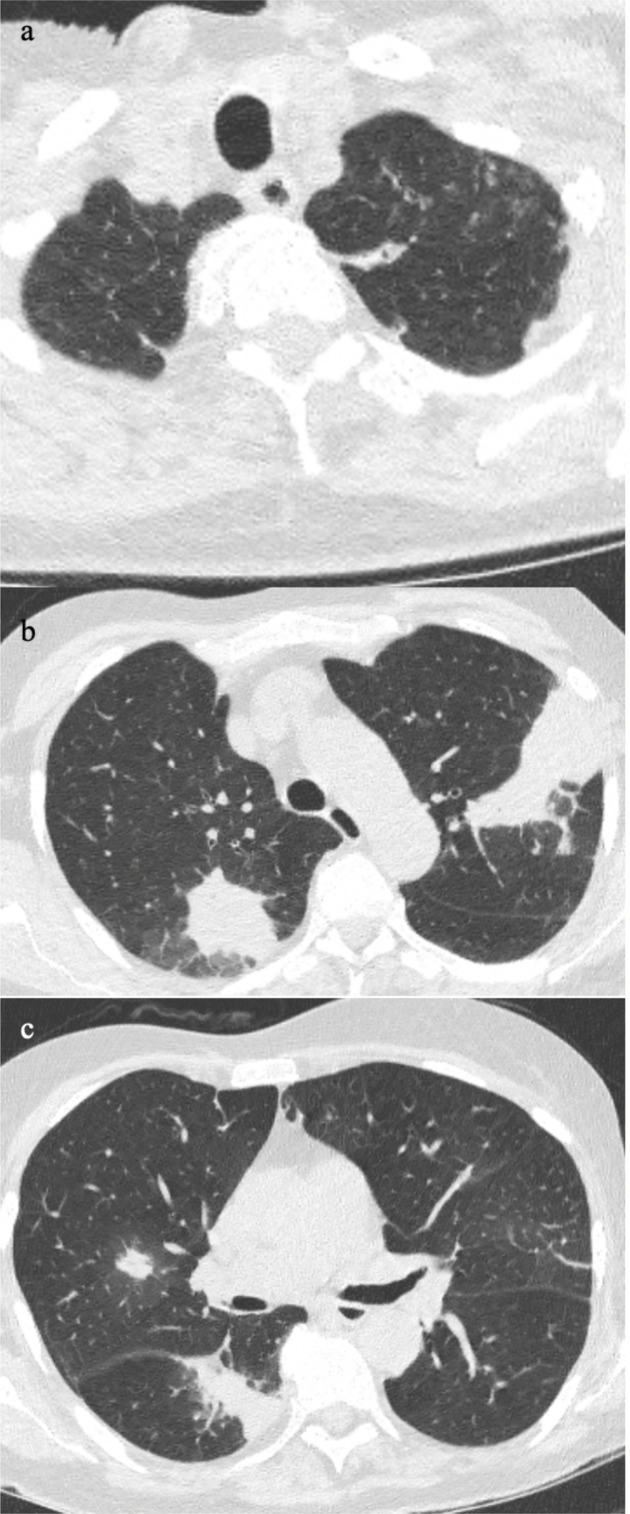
Patient has acute myeloid leukaemia and presented with neutropenic sepsis and cough. The chest CT showed mucus plugging and nodular ground glass in the left lung apex (7a), which is most likely due to bacterial infection. The mass-like consolidation in the rest of the lungs (7b-c) and a solid nodule with surrounding ground glass in the right upper lobe (7c) is more favourable of fungal infection.
